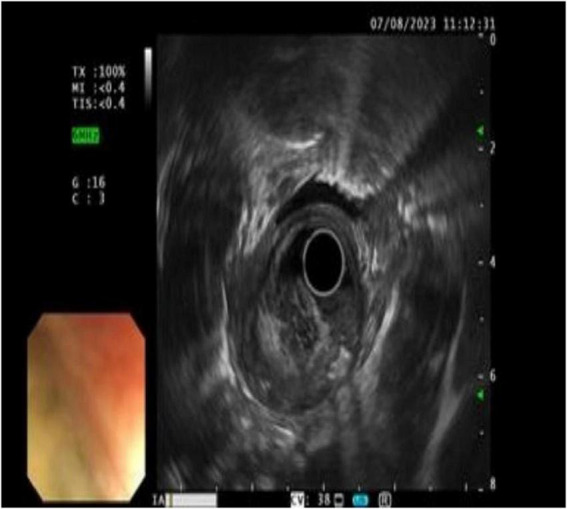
Ultrasound findings: the wall of the esophageal lesions was significantly thickened and hypoechoic, with hyperechoic and fluid anechoic areas in the wall, and some areas had blurred boundaries with the outer membrane.
